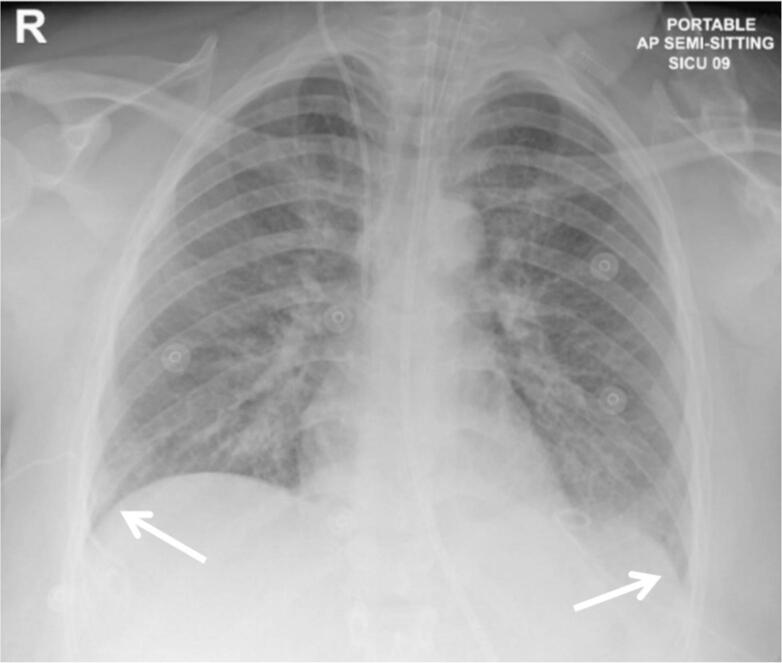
Chest x-ray after drainage of pleural effusion: No significant interval changes about the lung field. Both costophrenic angles are clearer in comparison with previous x-ray (white arrows). Bilateral chest drains in place.
